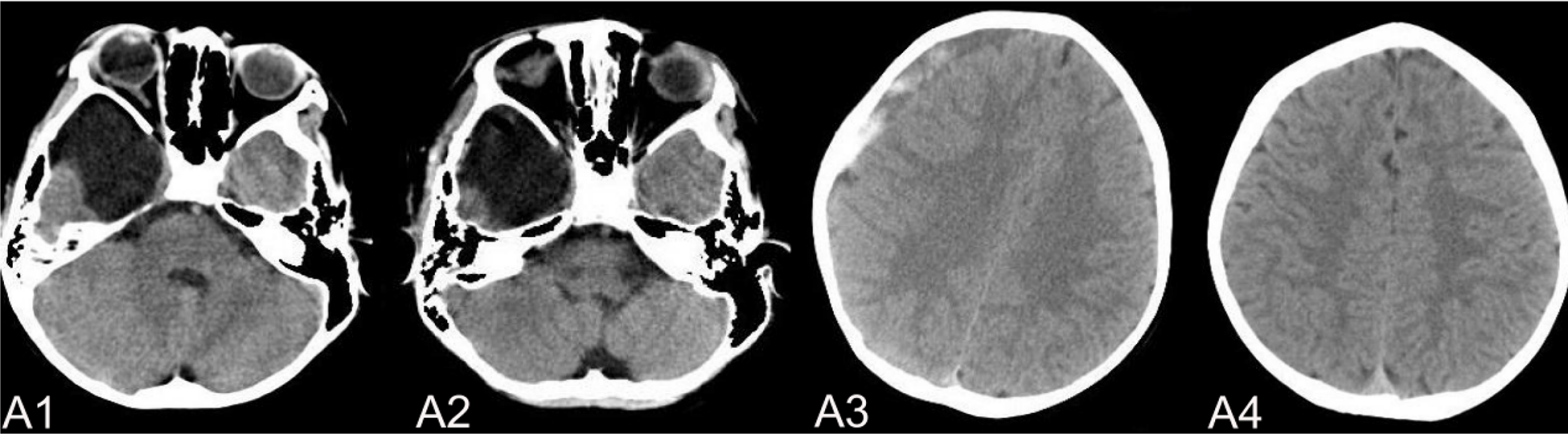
(A) shows a patient,who had a subdural hemorrhage during the follow-up time after operation. A1 and A2 shows the IAC CT images before and after operation. A3 shows the IAC hemorrhage after head trauma during the follow-ups. A4 shows the IAC hemorrhage disappeared after hemostasis treatment.
